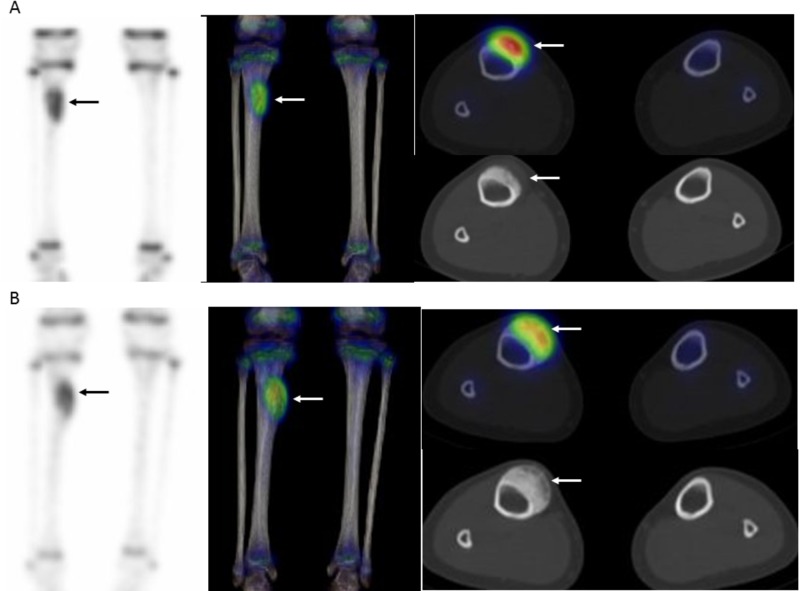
99mTc HMDP SPECT/CT of the tibial periosteal oseteosarcoma before and after neoadjuvant chemotherapy (NAC) A) Pre-NAC bone single photon emission computed tomography/computed tomography (SPECT/CT) (maximum intensity projection (MIP), coronal, and axial SPECT/CT) shows a focal and intense uptake of the mass; B) Post-NAC bone SPECT/CT (MIP, coronal, and axial SPECT/CT) shows the focal uptake with almost no change. ^99m^Tc HMDP: technetium 99m hydroxymethylene diphosphonate
